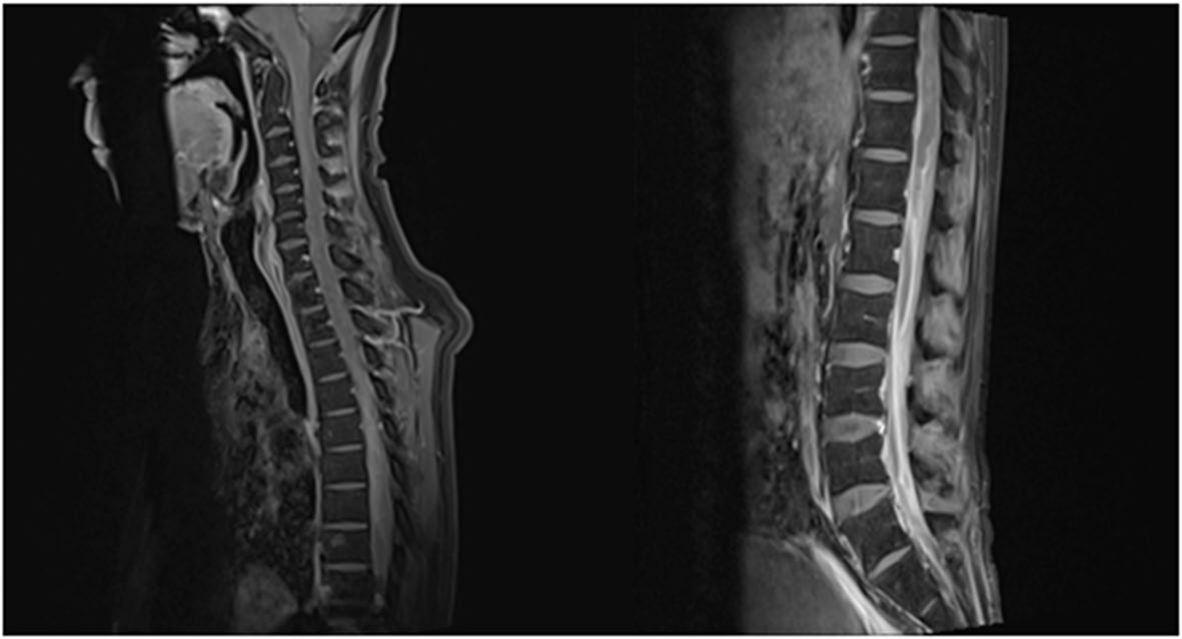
T2W sagittal image of the MRI pan spine revealed patchy central long segment T2 hyper intensity in the thoracic spinal cord extending from T6 level to conus medullaris
